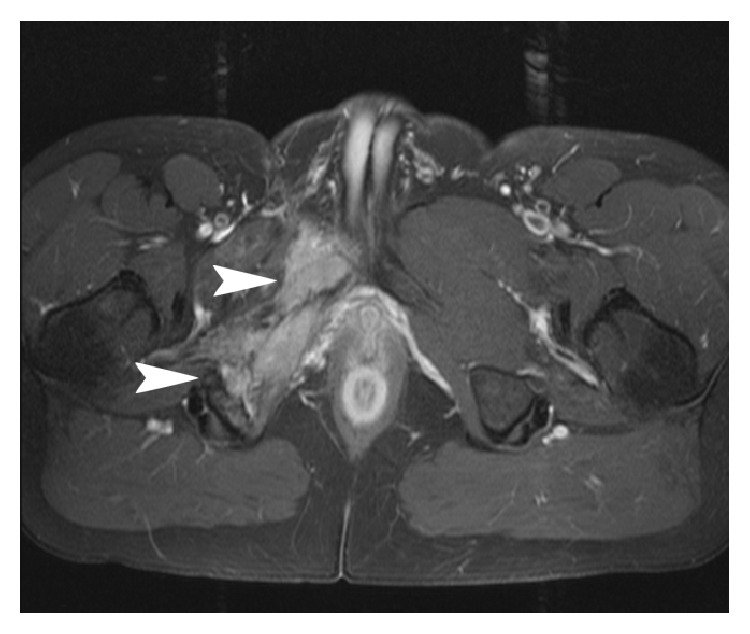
Gadolinium enhanced axial T1 CT (December 2005) of the pelvis with fat saturation demonstrates postsurgical change and irregularity of the right inferior pubic ramus, with an adjacent associated enhancing soft tissue mass (arrows).
